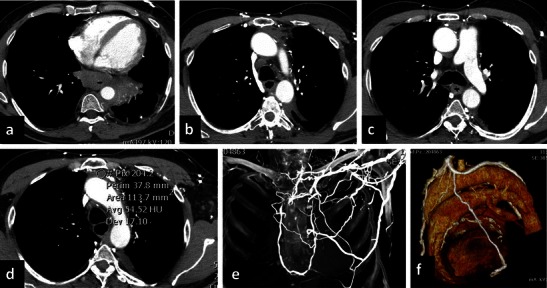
Radiation-induced fibrosing mediastinitis. A 58-year-old male treated 5 years previously with radiotherapy for mediastinal bulky lymphoma. The patient reported dysphagia, and a PET scan showed an uptake in the distal portion of the oesophagus. The CT scan shows a pathological thickening in the oesophagus and a parenchymal consolidation in the left lower lobe (a). Moreover, CT shows retrograde opacification of the azygos vein, hemiazygos vein (b, c) and aortic nipple. Increased density (d) is seen in the mediastinal tissue, particularly in the anterior mediastinum. Multiple collateral pathways were opacified, as confirmed by coronal MIP (e) and VR (f). These findings are consistent with fibrosing mediastinitis secondary to radiotherapy
